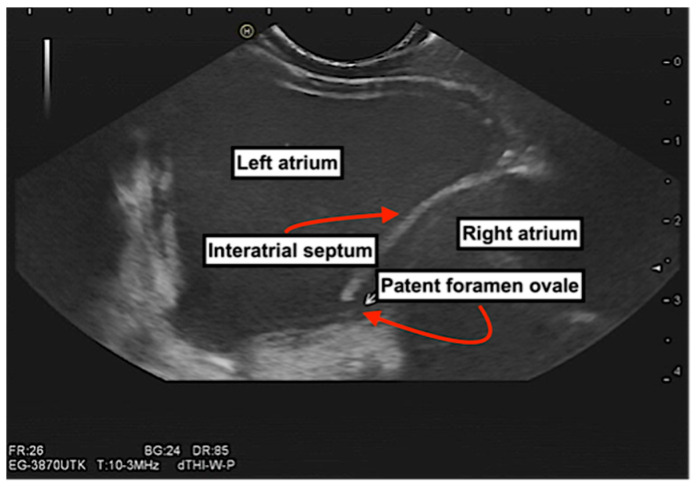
Endoscopic ultrasound demonstrated the morphology of left atrium in one view and the relation to right atrium. Patent foramen oval is incidentally detected.
